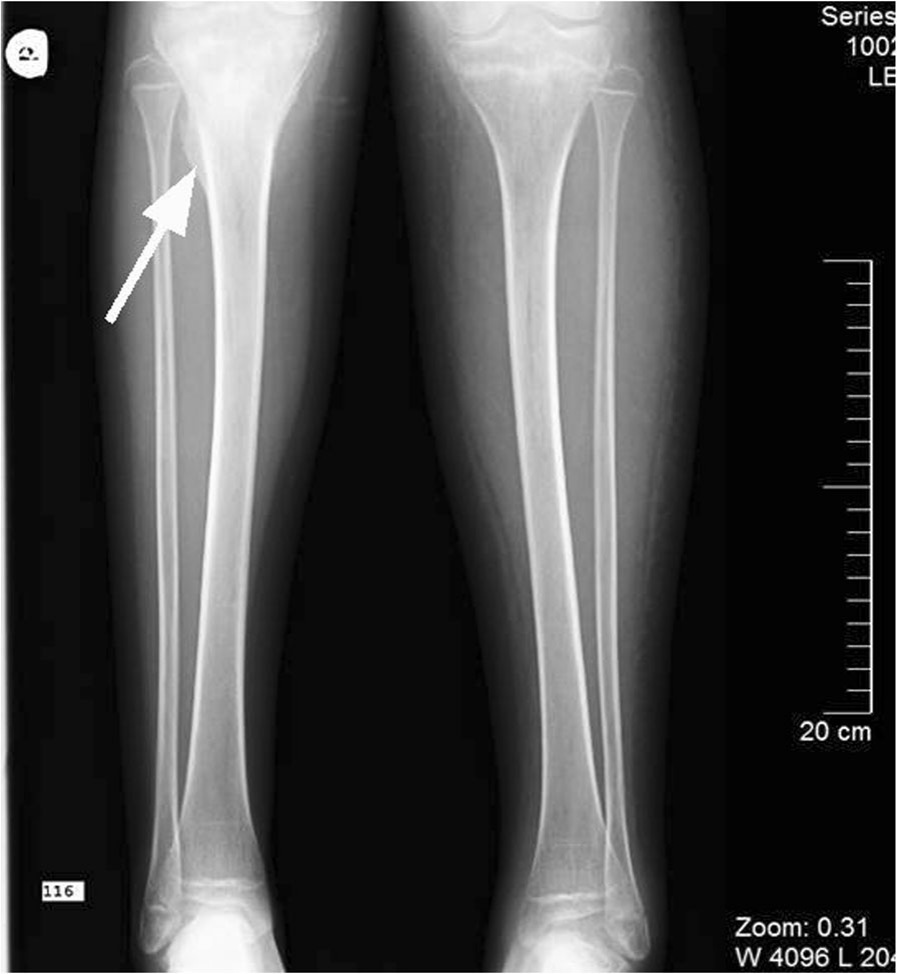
X-ray of the legs.Hyperostosis on the left tibia metaphysis is indicated by a white arrow
